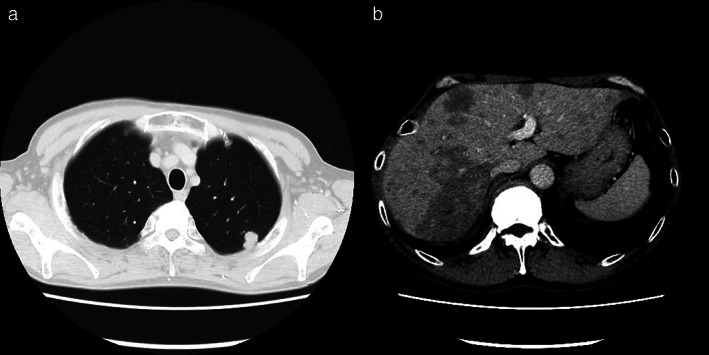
Pretreatment computed tomography (CT) findings. (a) A nodular shadow in the left upper lobe. (b) Multiple low‐density areas in the liver
